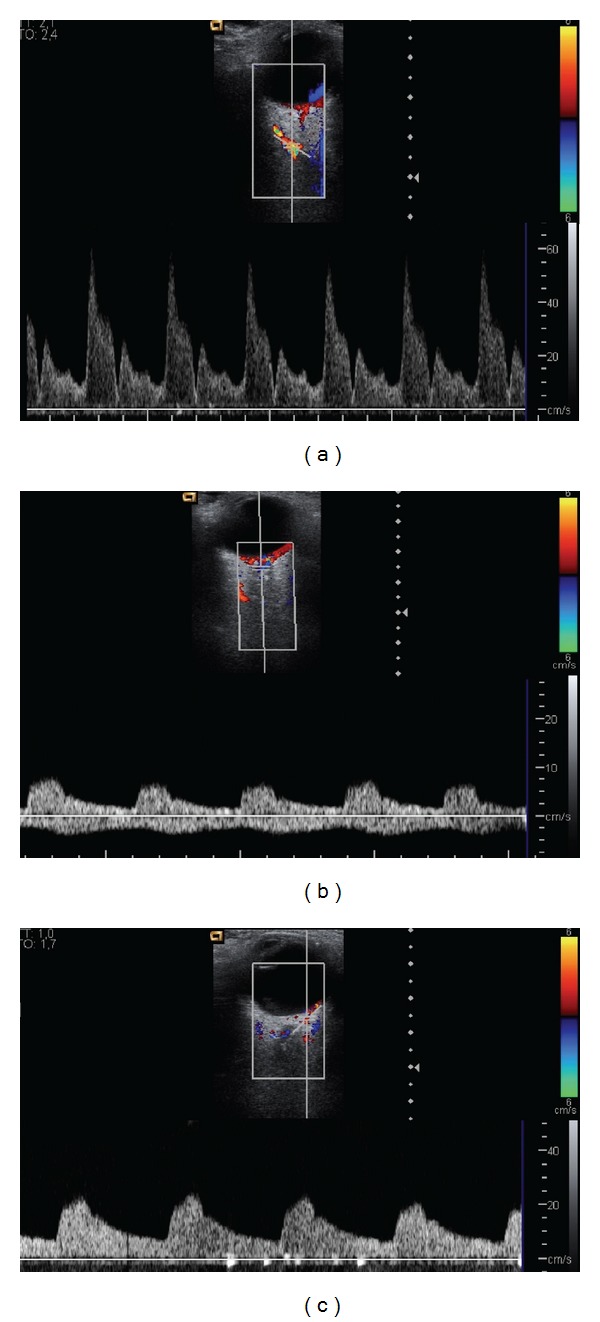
(a) Transverse color Doppler image that demonstrates the location of the ophthalmic artery (OA), medial to the optic nerve (ON), and the typical OA waveform obtained by pulsed Doppler that shows a sharp systolic peak, a dicrotic notch, and a relatively little flow in diastole. (b) Transverse color Doppler image showing the central retinal artery (CRA) and central retinal vein (CEV) in the center of the ON. The typical waveforms for central retinal vessels show the CRA curve above the zero axis (with rounded systolic peak and continuous flow during diastole) and the CRV below the zero axis (with low and continuous flow). (c) Transverse color Doppler image that demonstrates the posterior ciliary arteries (PCSAs) in the retrobulbar fat. The typical PCAs waveform obtained by pulsed Doppler shows a blunted systolic peak and a low to moderate flow velocity during diastole.
